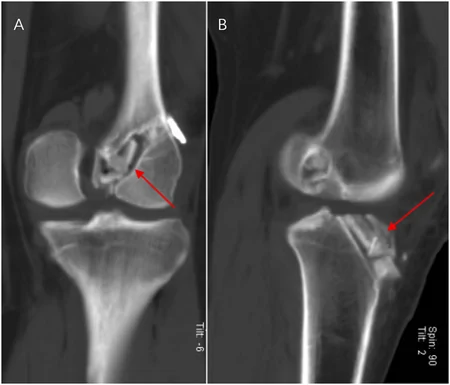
Postoperative CT evaluation of femoral and tibial tunnels. Postoperative CT of right knee in supine position. (A) Femoral tunnel showing complete graft impaction and restored diameter (arrow indicates tunnel). (B) Tibial tunnel showing full filling with autologous bone and restored morphology (arrow indicates graft location). Orientation: sagittal and coronal planes; arrows highlight grafted tunnels. (CT, computed tomography.)
